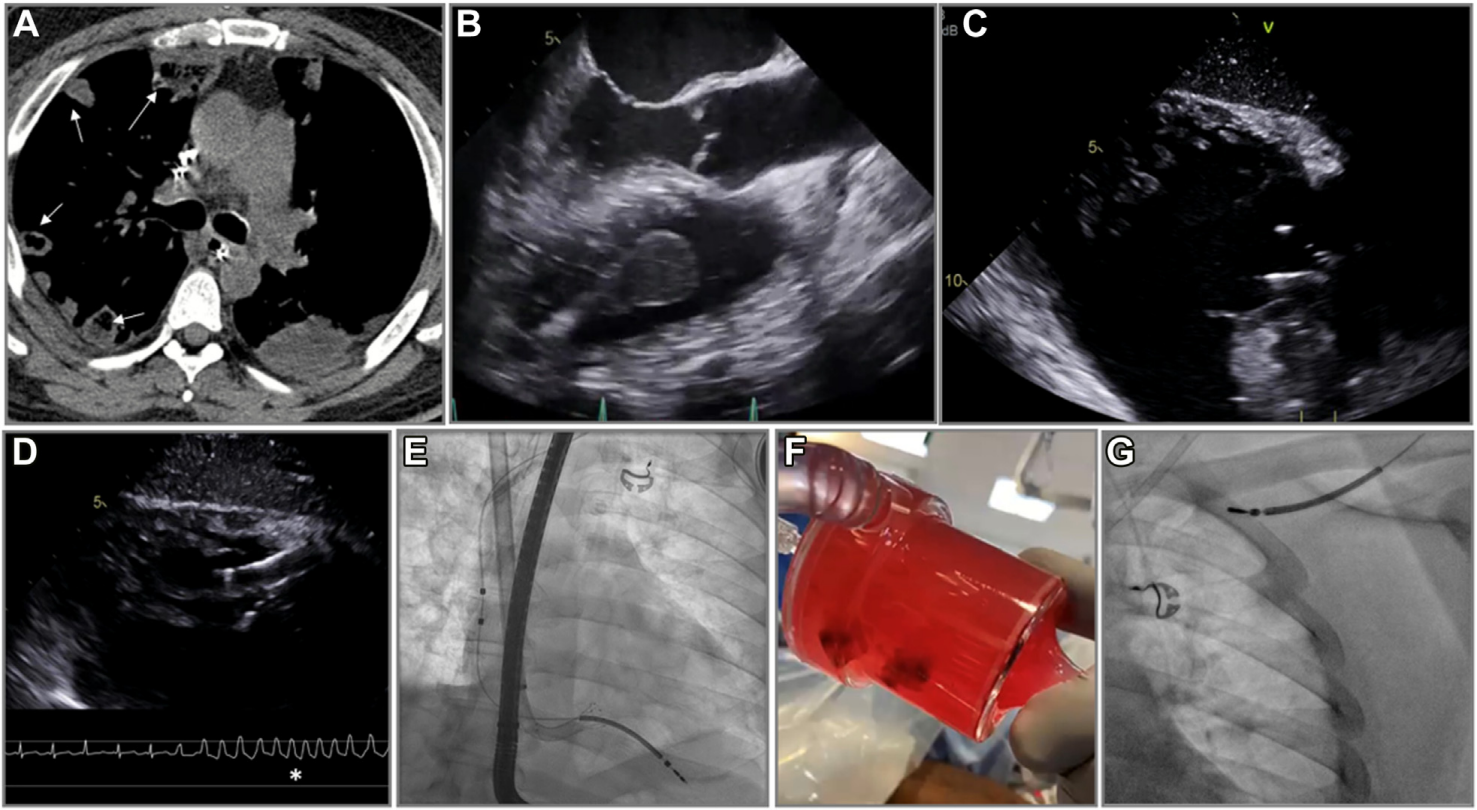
Percutaneous mechanical aspiration in cardiac implantable electronic device related infective endocarditis. Patient presented with recurrent pulmonary septic emboli despite antmicrobials (A, white arrows). There was a large vegetation on the right ventricular lead (B). Patient underwent percutaneous mechanical aspiration using AngioVac under transesophageal (C,D) and fluoroscopic (E) guidance, with a run of ventricular tachycardia due to a suck down event that resolved with repositioning the catheter (D). The vegetation was successfully aspirated (F) and led to safe lead extraction (G).
